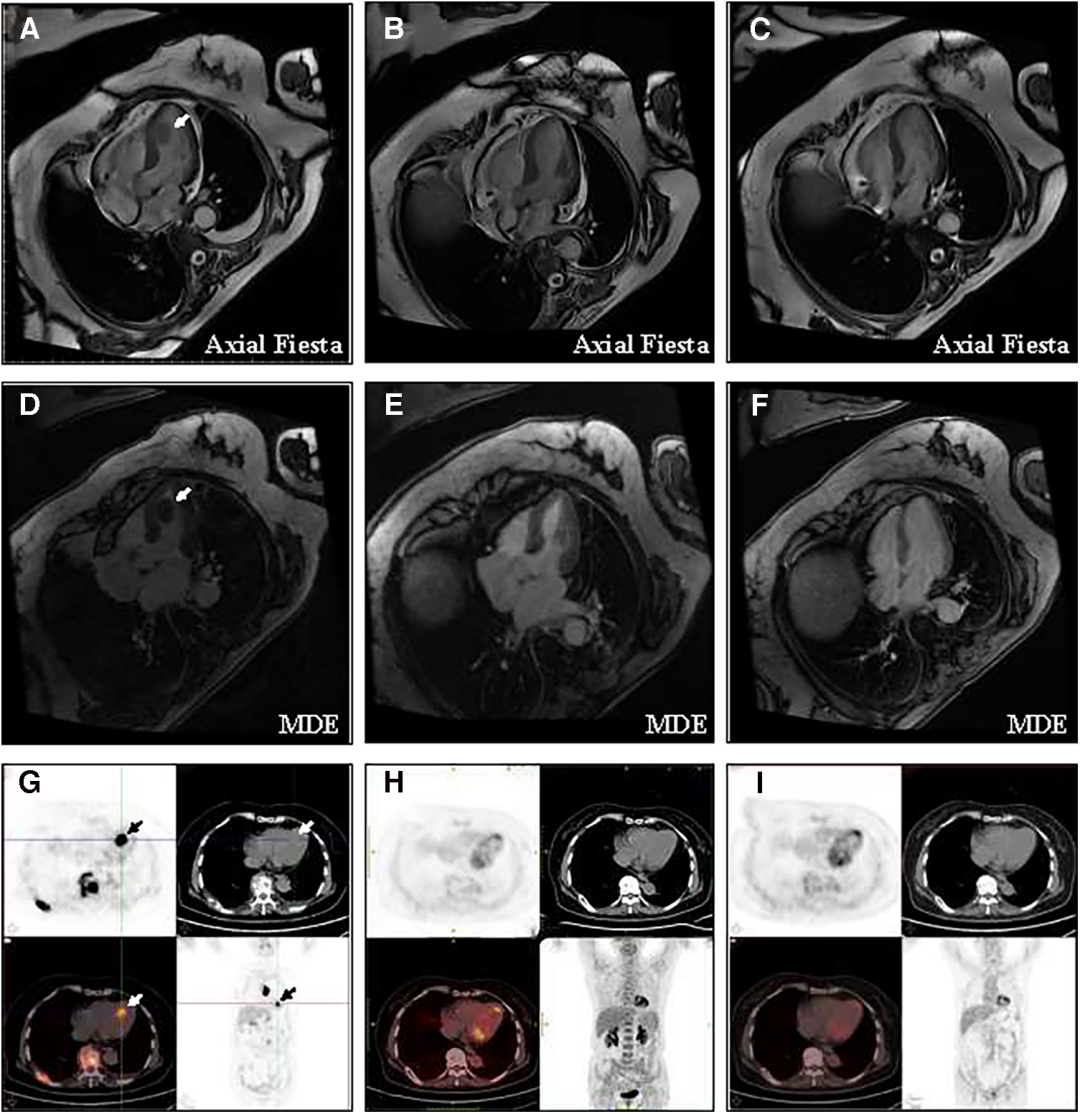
Change of the left ventricular mass at baseline and after treatment (A) CMR showed that the baseline size of the mass is 1.6 cm × 1.1 cm × 2.1 cm. (B) CMR showed that after 4 cycles of R-CHOP, the tumor at all sites had almost complete regression. (C) CMR showed no recurrence of cardiac mass in the five years of follow-up. (D) Long-axis four-chamber myocardial delayed enhancement (MDE) showed that the left ventricular mass appeared non-gadolinium enhancement at baseline. (E) After 4 cycles of R-CHOP treatment, long-axis four-chamber MDE showed the left ventricular mass had almost complete regression. (F) MDE showed no recurrence of cardiac mass in the five years of follow-up. (G) PET/CT showed that the maximum standardized uptake value for left ventricular masses was 16.5 and 19.7 after delay. (H) After 4 cycles of R-CHOP treatment, the left ventricle mass had almost complete regression and the FDG uptake of the left ventricular had returned to normal levels. (I) PET/CT showed no recurrence of cardiac mass in the five years of follow-up.
